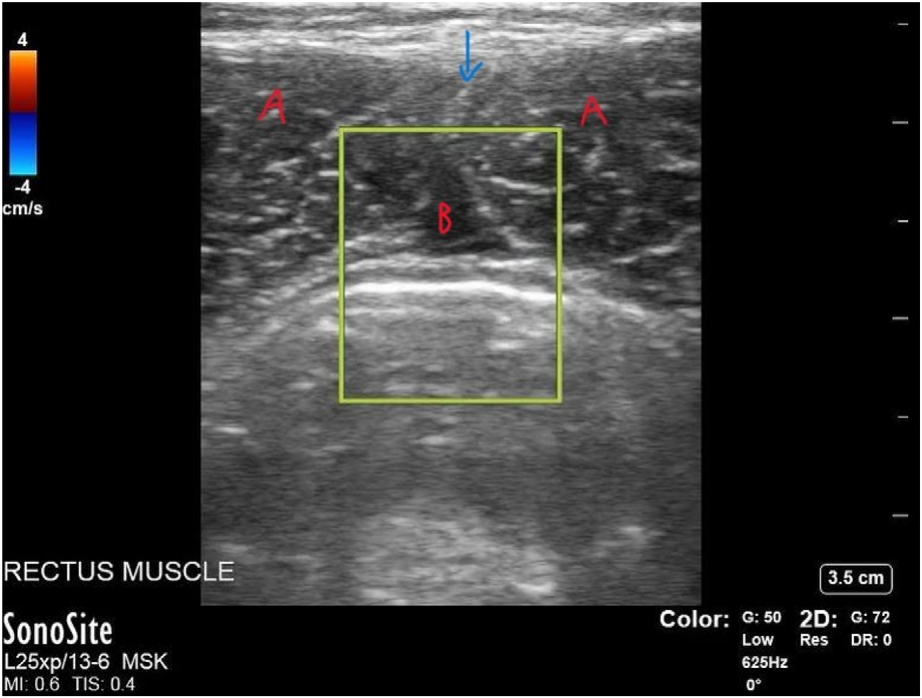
Point-of-care ultrasound of the rectus abdominus in short-axis view demonstrating a small, 1 × 2-centimeter collection of fluid. In the near field is skin and subcutaneous tissue. The hyperechoic midline stripe between the two triangular rectus abdominis muscle bellies (“A”) on either side is the linea alba (blue arrow). The hypoechoic area (labeled “B”) between the rectus abdominis muscles and below the linea alba is likely edema. The yellow rectangle is a color flow application, which indicates arterial (red) or venous (blue) blood flow. In this case, there is no flow, which is expected in the case of edema.
